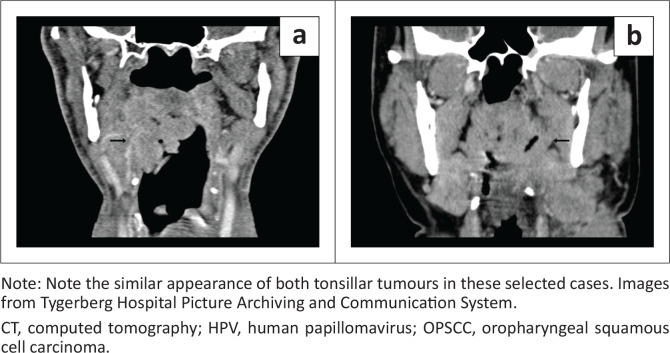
Coronal contrast enhanced CT scan showing a tonsillar tumour in (a) a HPV-positive OPSCC and (b) a HPV-negative OPSCC patient.
